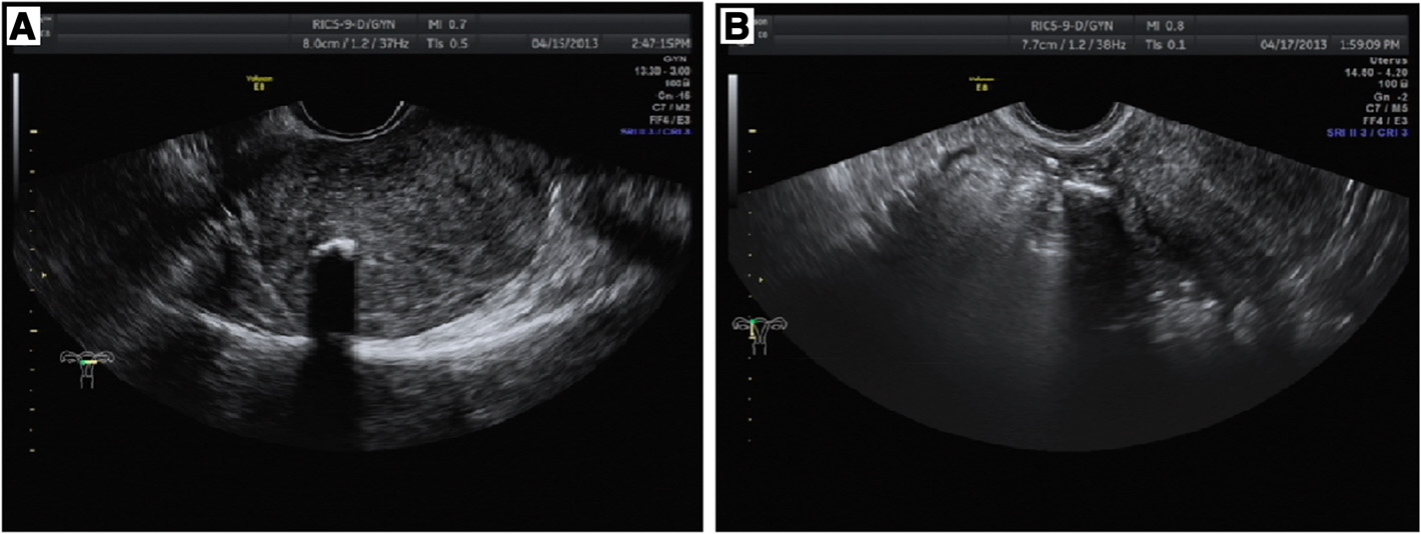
Two strong echogenic stripes with attenuation in the uterine submucosa (A) and mesosalpinx (B) in the transvaginal ultrasonography, respectively.
